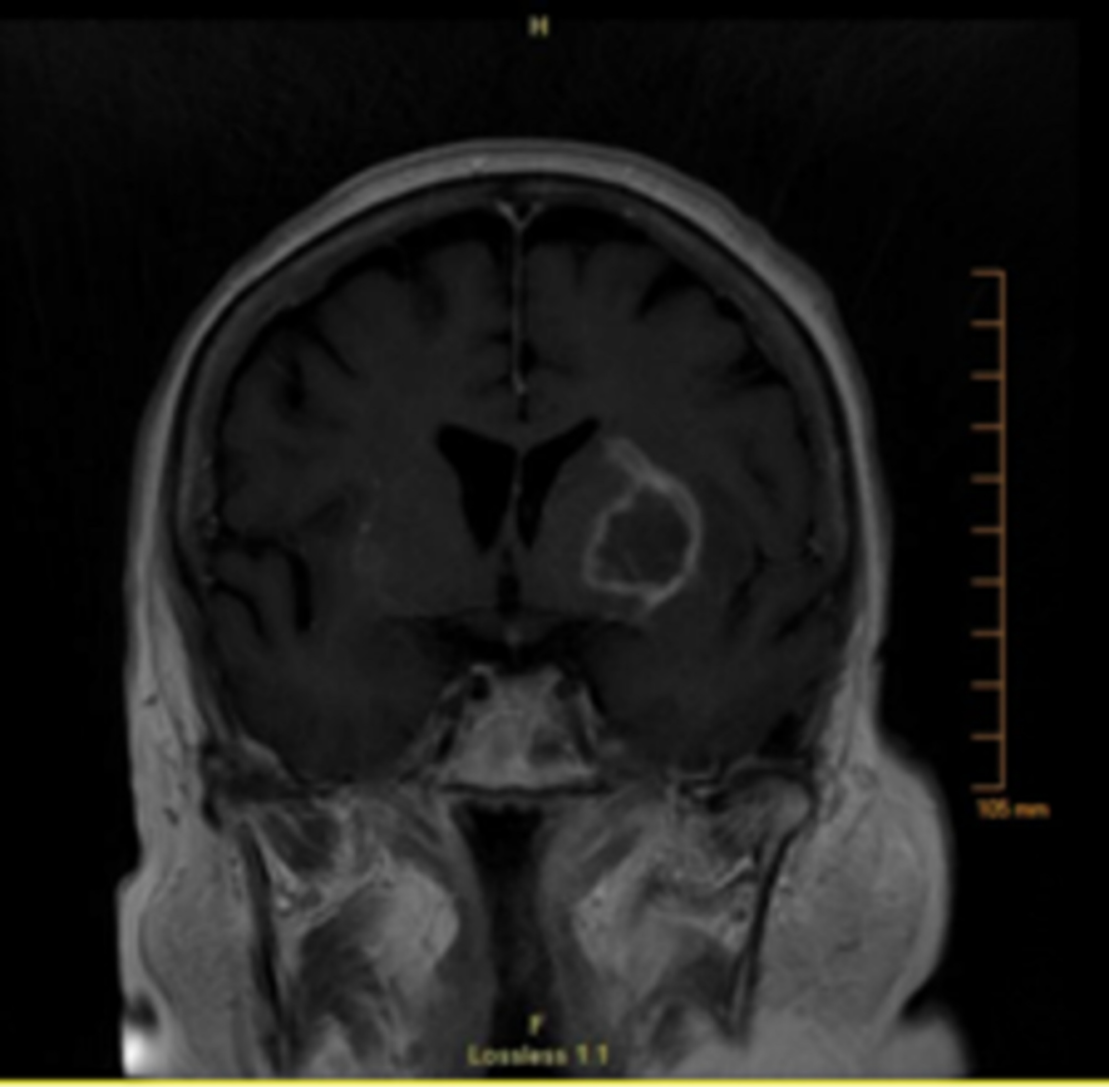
MRI of the brain (T1 fluid-attenuated inversion recovery) - view 2 The imaging revealed a 4 x 2 cm ring-enhancing in the basal ganglia on the left with a small enhancing lesion in the caudate nucleus as well as a small 1 cm round ring-enhancing lesion in the putamen on the right. There were surrounding vasogenic edema and mild mass effect on the left lateral ventricle MRI: magnetic resonance imaging
